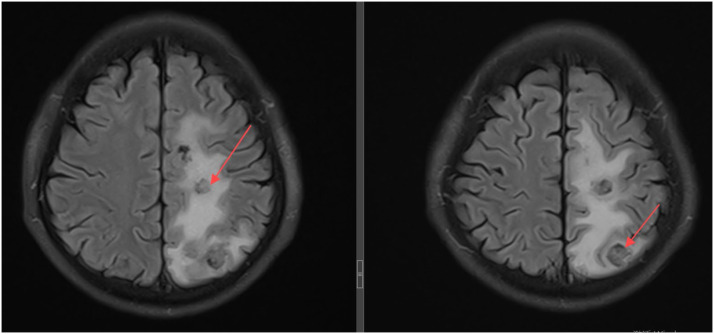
Neuroimaging demonstrating lesion progression and worsening edema. As indicated by the red arrow, prior to initiating anti-TB therapy, MRI revealed multiple ring-enhancing lesions with marked perilesional edema.
